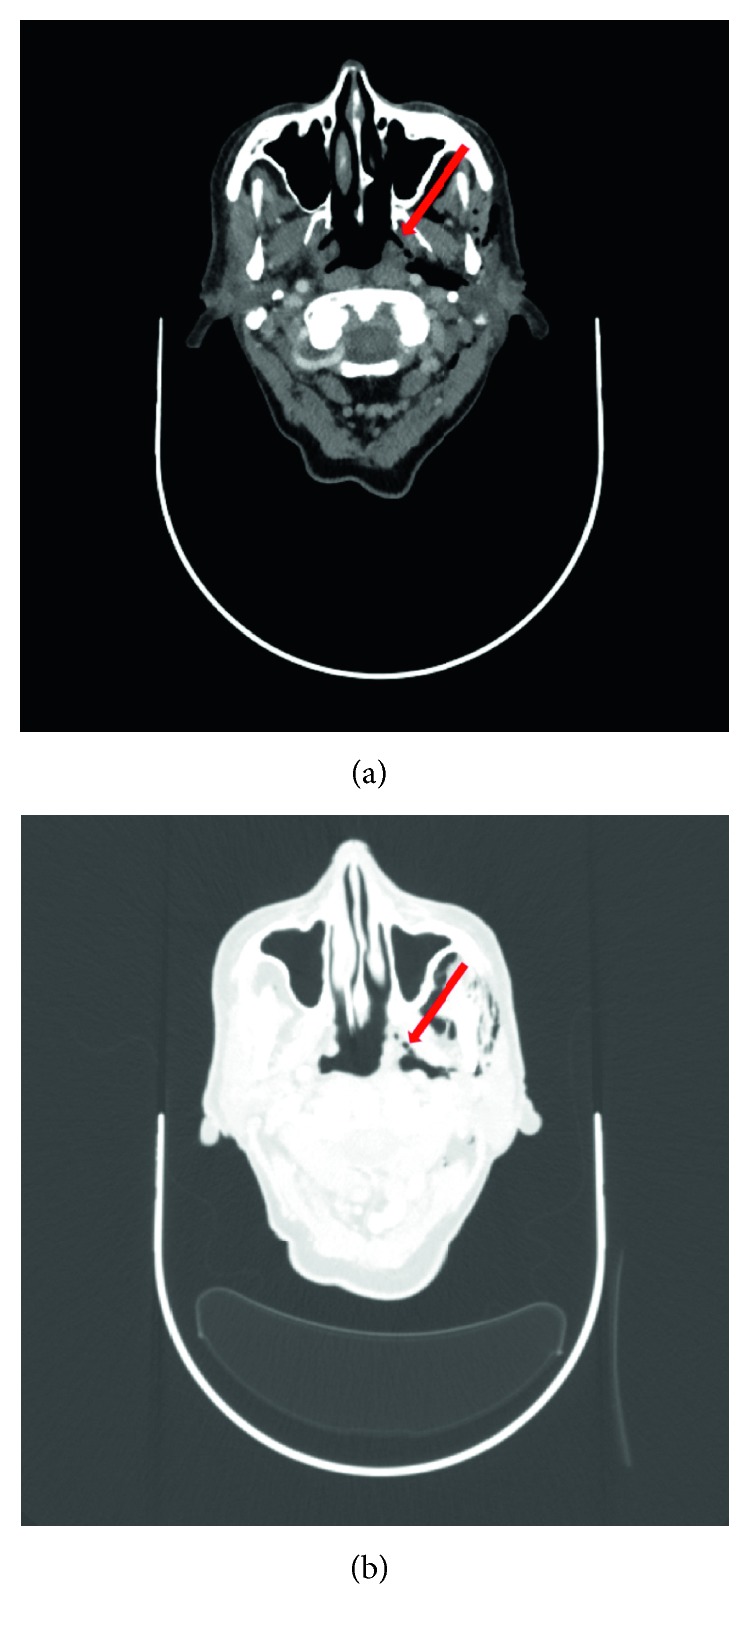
(a) Axial postcontrast CT at the level of the fossa of Rosenmüller demonstrating the site of perforation through the left nasopharynx (arrow). (b) Axial postcontrast CT below the level of the fossa of Rosenmüller demonstrating air tracking through the left parapharyngeal space (arrow) and around the deep and superficial parotid lobes.
